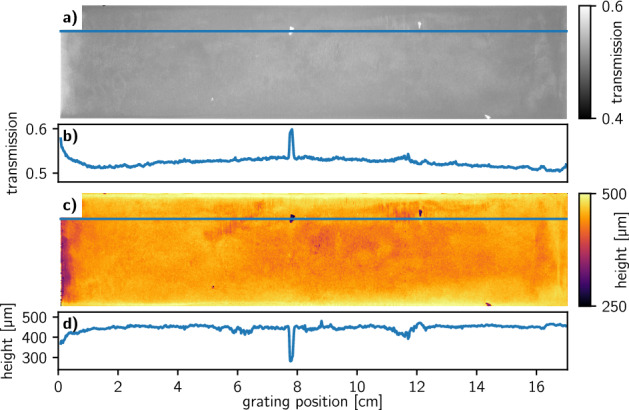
Angular X-ray transmission analysis results for the 170 mm × 38 mm sized grating filled with the bimodal W particle mixture. (a) Transmission image that has been virtually recorded with a parallel beam geometry and (c) geometrical height image. Corresponding line plots along the blue lines are given in (b) and (d).
